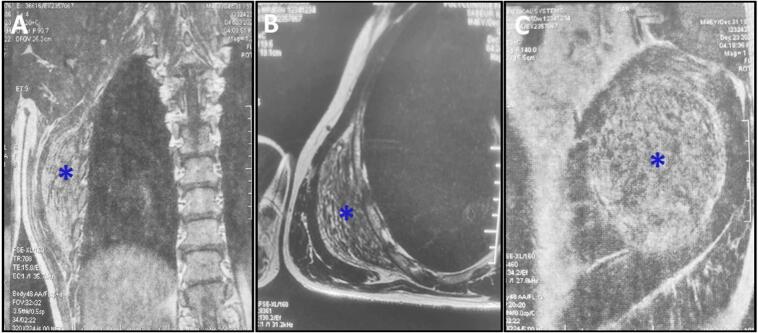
A,B, C: Magnetic resonance imaging depicting an elongated mass (blue asterisk) located in the right posterolateral thoracic wall, adjacent to the lower angle of the scapula. The mass (blue asterisk) displayed well-defined borders and a striated pattern, attributed to alternating linear structures with low signal intensity on T1 and T2 MRI sequences within a fatty background.
